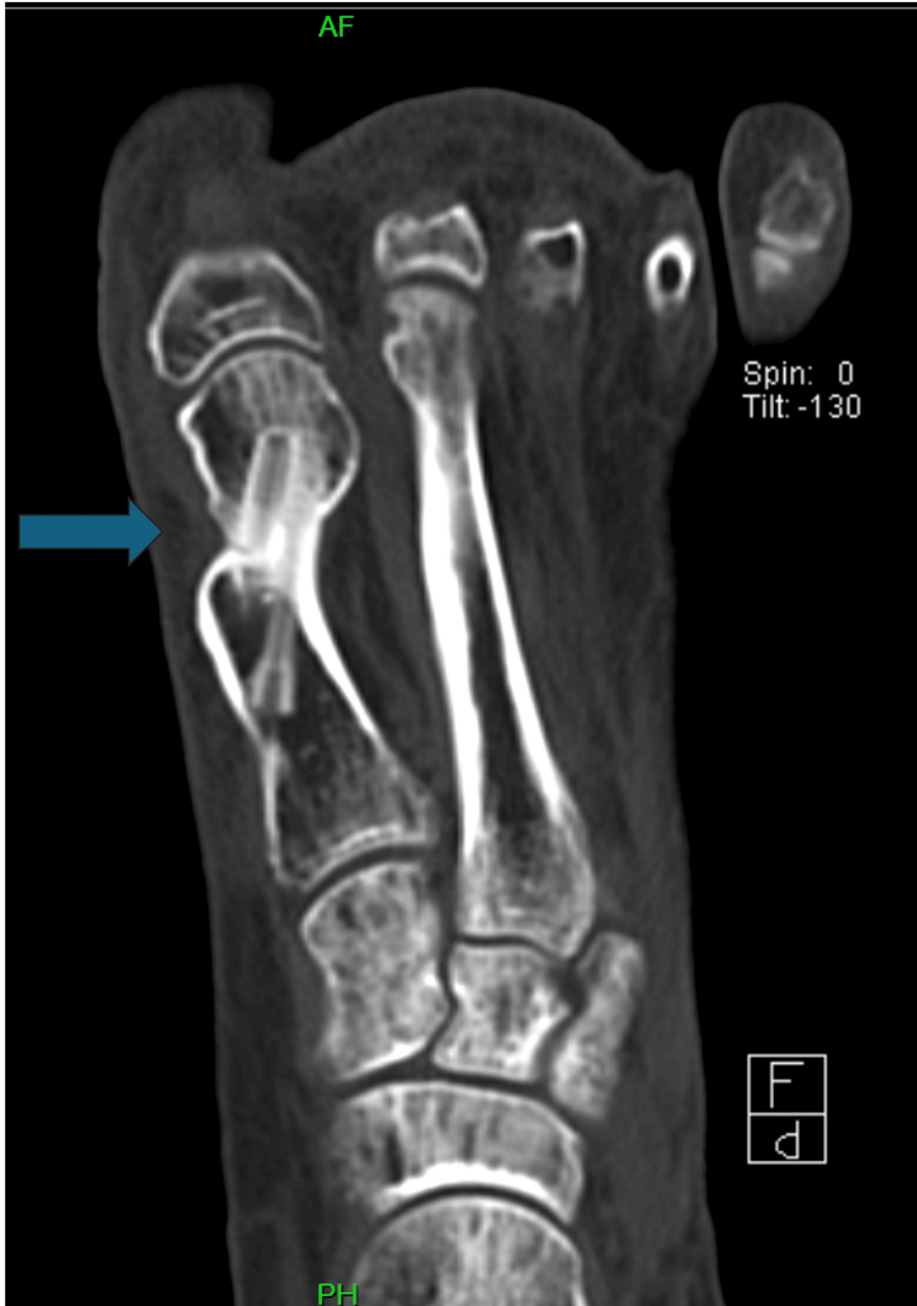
Coronal CT image confirming osseous union at three months post-revision (blue arrow), indicating the site of bone healing
